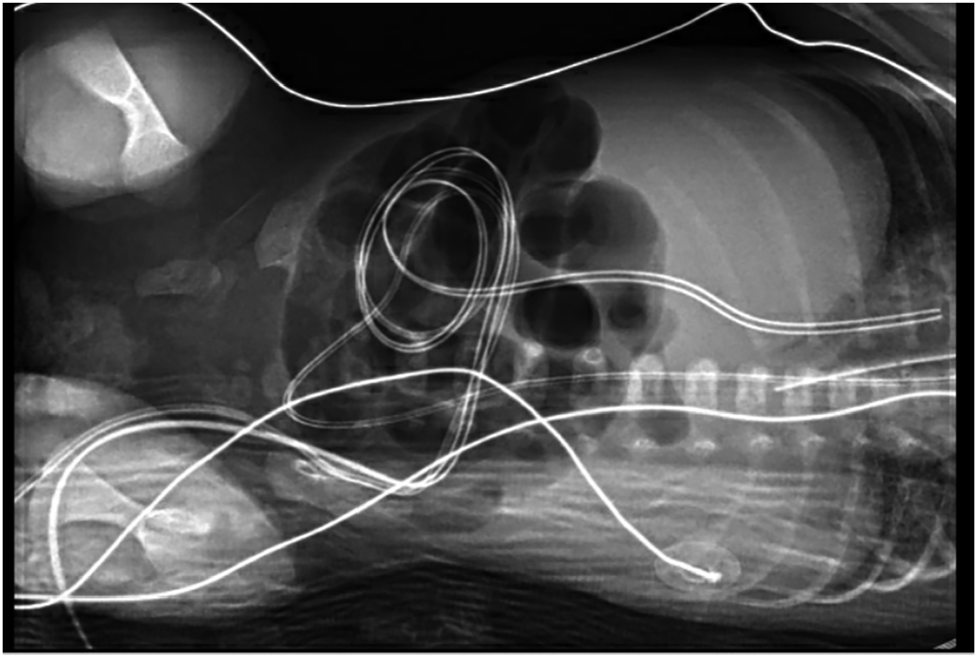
Radiographic imaging revealed that there is no evidence of free air in the peritoneal cavity. There was no evidence of portal physical exam findings consisted of generalized edema that seemed to worsen over the next five days. On DL 8, she developed hemolytic uremia syndrome (HUS) with a right grade three hemorrhage and left grade two hemorrhage in the cerebral ventricles and mild prominence of the right lateral ventricle (Figure 2). Her worsening edema manifested itself as a round and full abdomen and a rounded head. Abdominal ultrasound on DL 9 found trace ascites and pelviectasis venous gas.
